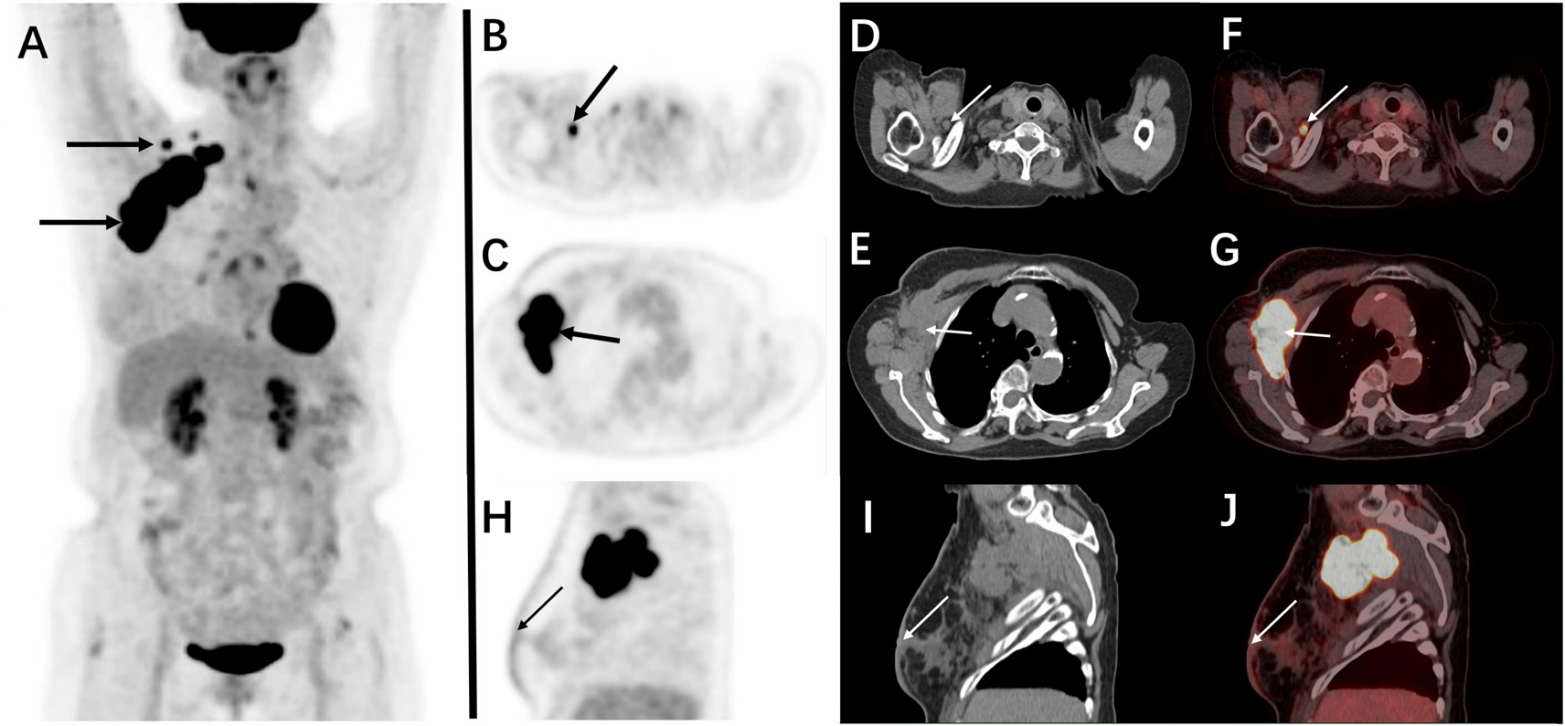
(A) A three-dimensional maximum intensity projection reconstruction of the PET images is seen and shows abnormal FDG uptake in the primary malignancy in the right chest wall; the SUVmax was 23.8. (B) Axial PET images showing the subclavian lymph nodes showed emission concentration; (C) radioactivity was concentrated in the chest wall and axillary lymph nodes; and the tumor developed metastasis. (D, E) The corresponding lesions on non-contrast CT. (F, G) The fused PET/CT images. (H) Sagittal PET images: increased size of the right breast, skin thickening, dense glands, and slightly increased metabolism; the SUVmax was 2.3. (I) The corresponding lesions on non-contrast CT. (J) The fused PET/CT images.
